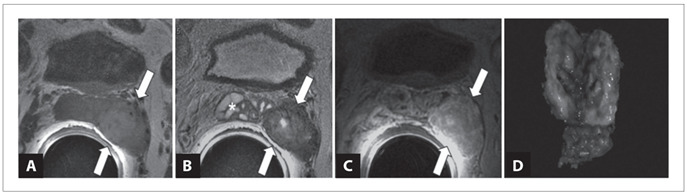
Paraganglioma of seminal vesicle seen on endorectal magnetic resonance imaging. A) T1-weighted axial image showing a slightly hyperintense solid mass in the left seminal vesicle (arrows).The presence of focal hyperintense areas within the mass is due to the hemorrhagic component of the lesion; B) T2-weighted axial image demonstrating that the mass (arrows) is predominantly hypointense. Note the normal appearance of the right seminal vesicle (*); C) Contrast enhanced, T1-weighted axial image showing that the lesion (arrows) presents a high degree of contrast enhancement. D) Macroscopic appearance: seminal vesicle with a brownish, well-circumscribed smooth nodule measuring 30 mm.
